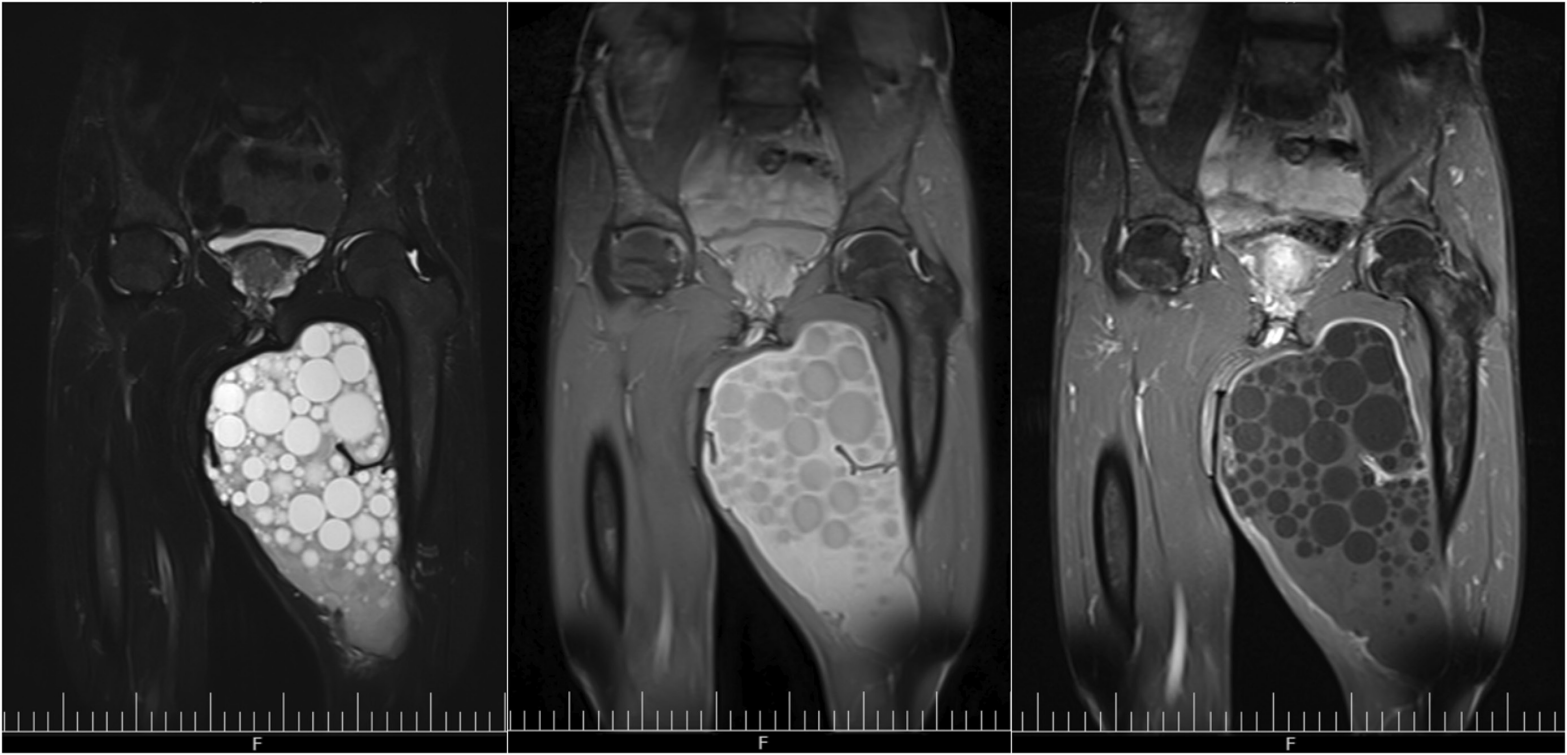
MRI findings of the cyst show numerous daughter cysts in a lobulated cyst in the left thigh
